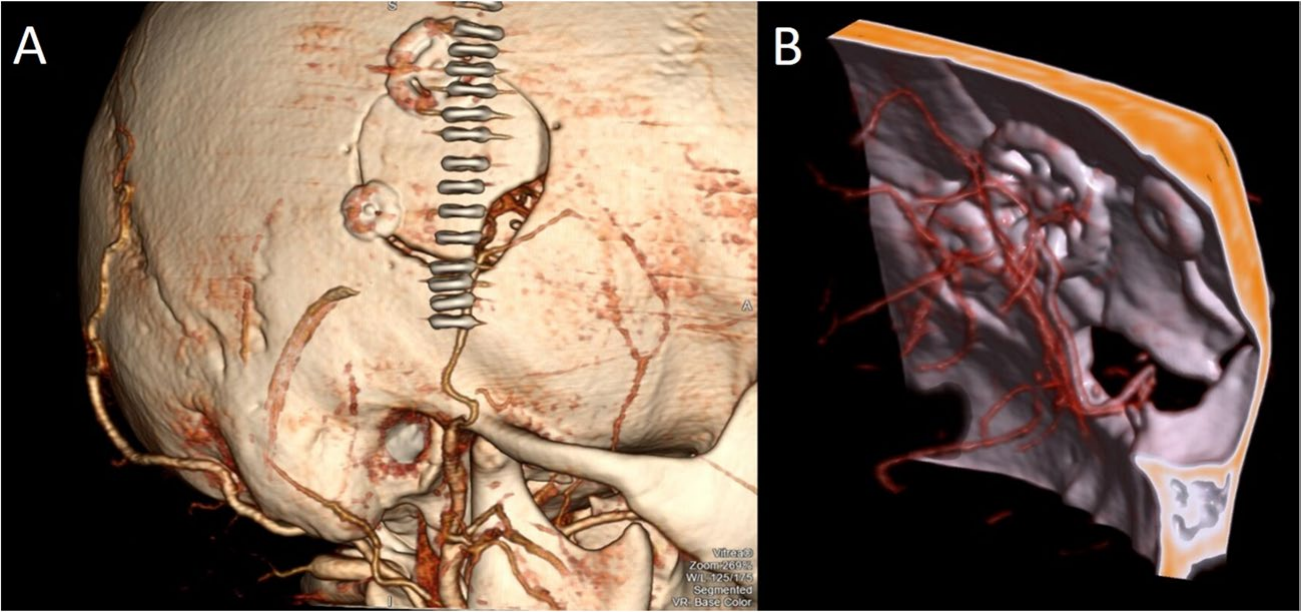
Bypass patency is evaluated in thin slice CT angiography with the aid of 3D rendering techniques (A & B) 3D, three dimensional; CT, computed tomography
